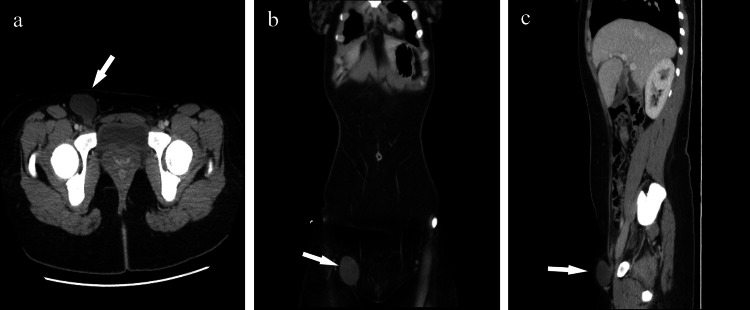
CT scan of the abdomen and pelvis showing the location of the hydrocele. Three CT scan images depict the hydrocele of the canal of Nuck (white arrows) in the right groin through (a) horizontal, (b) coronal, and (c) sagittal sections.
